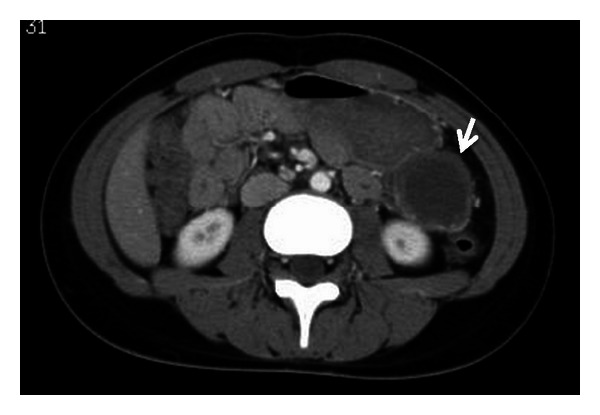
CT at three months after rupturing tumor. A cystic tumor is shown (arrow), protruding caudally from the pancreatic tail. The fluid around tumor had disappeared.
